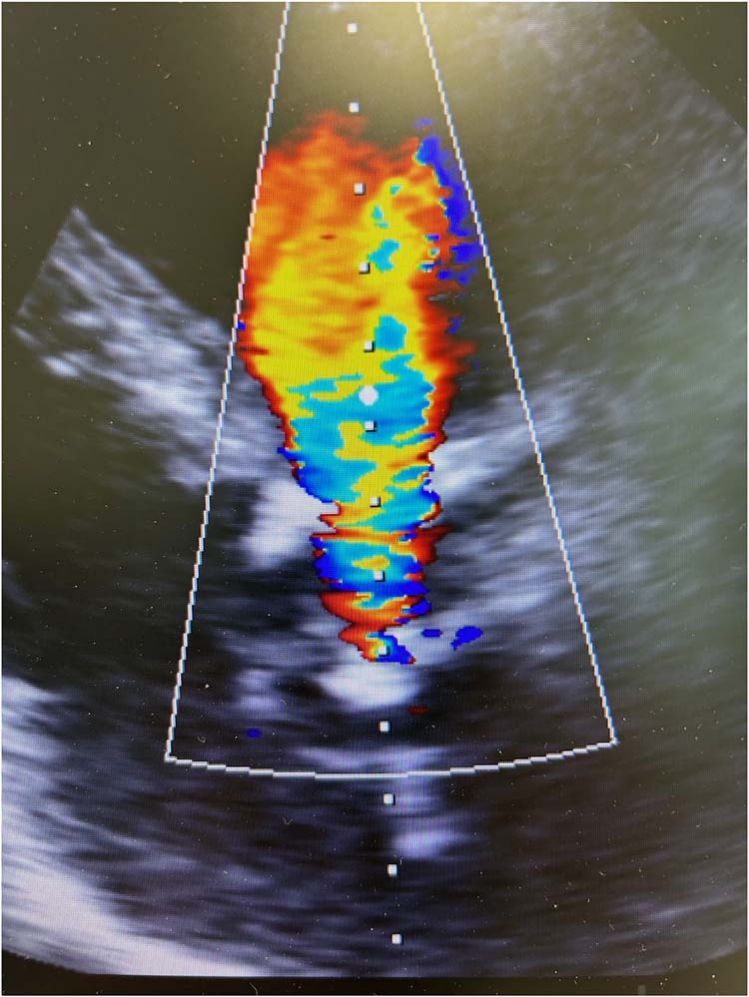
Preoperative transesophageal echocardiography showing significant regurgitation and stenosis of the aortic valve bioprosthesis.
